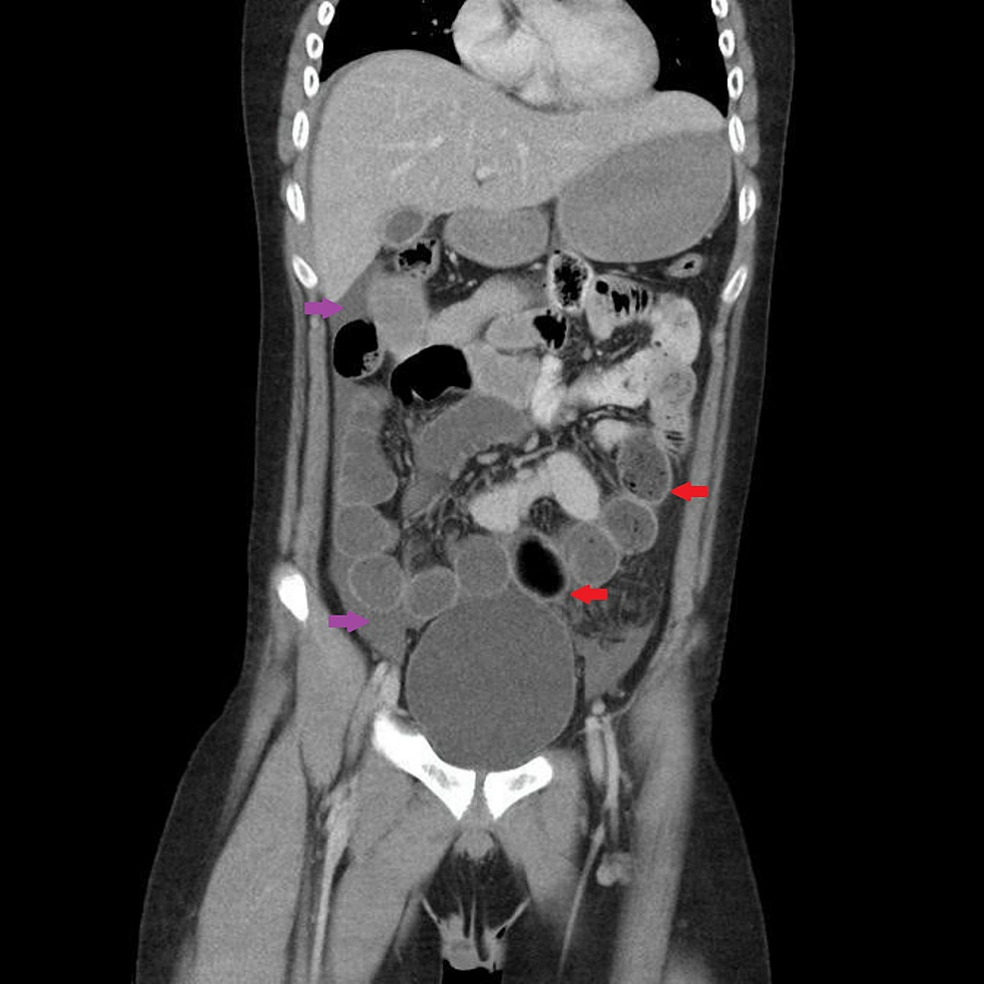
Abdominal computed tomography scan with contrast showing free fluid (purple arrows) around the liver, in the right iliac fossa, and pelvis, with dilated small bowel loops (red arrows).
